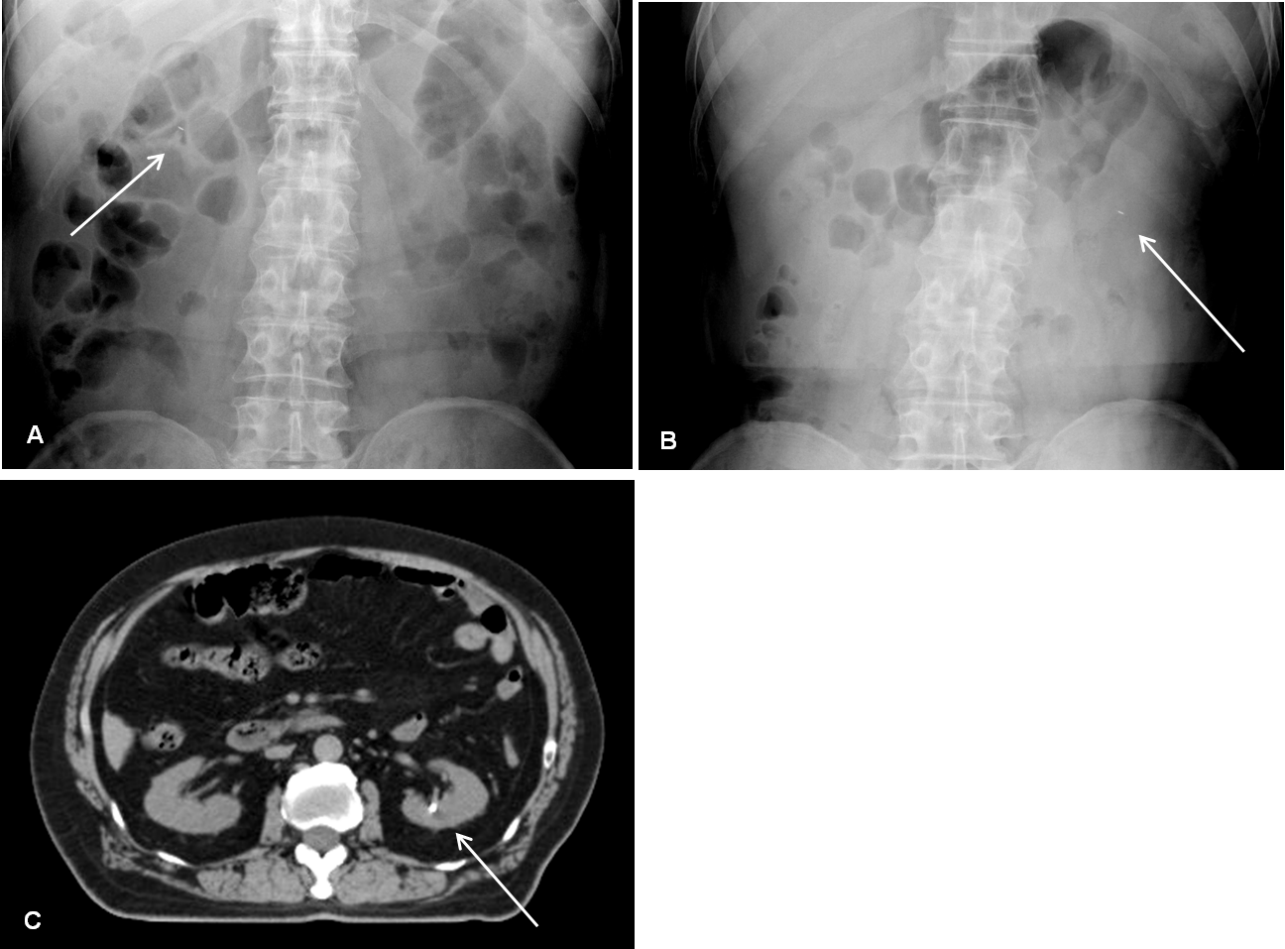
Case 1: Relocation from the right side of the middle abdomen to the left kidney. One day after seed implantation, a follow-up abdominal radiograph showed that a seed had migrated to the right side of the middle abdomen (solid arrow) (A). Two weeks later, the seed had relocated from the right side of the middle abdomen to the left side of the middle abdomen (solid arrow) (B). Subsequent computed tomography showed that the seed had migrated to the left kidney (solid arrow) (C).
